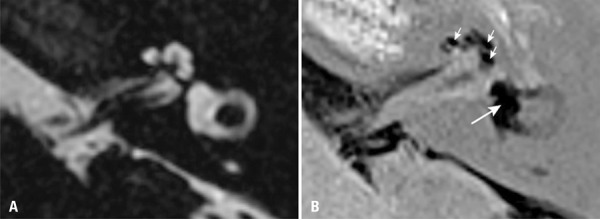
Axial magnetic resonance imaging of the left ear of a patient diagnosed with Ménière disease. (A) Highly T2-weighted sequence shows the labyrinthine fluid space (sum of endolymphatic and perilymphatic spaces). (B) Inversion recovery turbo spin echo with real reconstruction (3D real-IR) sequence 4 hours after intravenous gadolinium administration shows distension of saccule and utricle, which occupy most of the vestibular area (long arrow), and significant distension of cochlear duct (short arrows)
